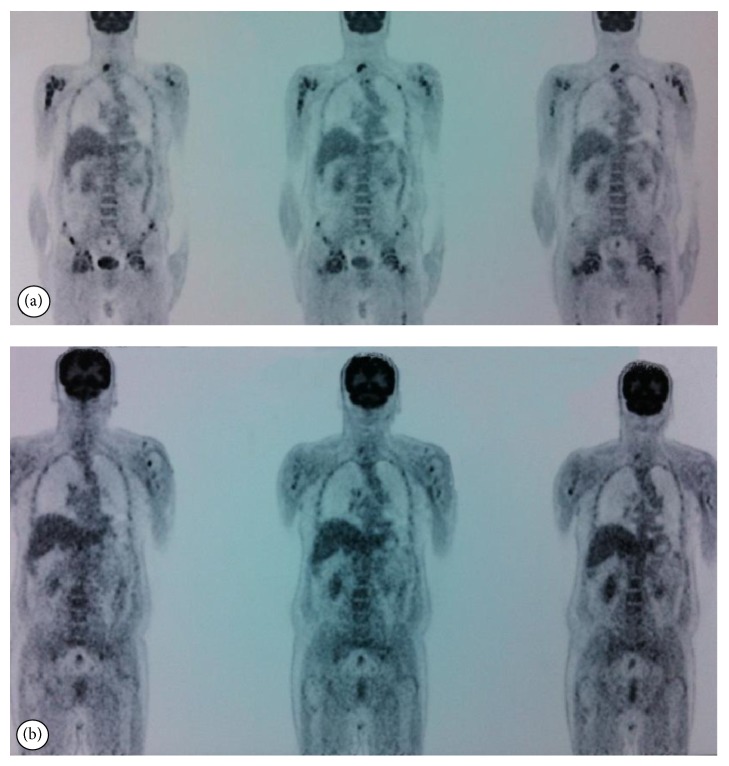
PET scan at diagnosis and 8 weeks after chemotherapy. (a) There was increased FDG uptake on axial skeleton, pelvis, and both femurs at diagnosis. (b) Follow-up PET scan demonstrated marked metabolic response with decrease in uptake of multiple metastatic lesions on both proximal humeri, the right lamina of C6 vertebra, and both first ribs.
